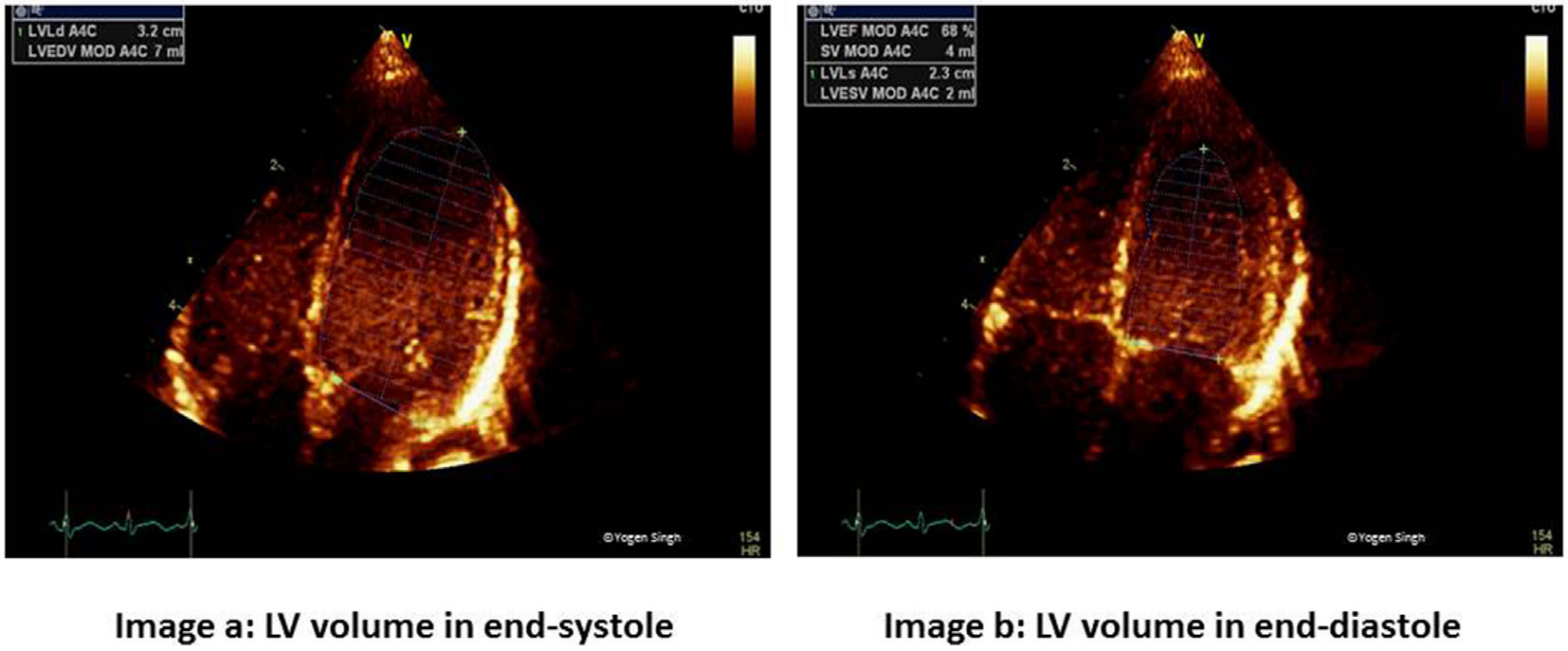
Simpson’s biplane method for measurement of ejection fraction (EF). Image a shows left ventricular end-diastolic volume (LVEDV) and image b shows left ventricular end-systolic volume (LVESV) measurements in apical four-chamber view to calculate EF. Similar measurements are done in apical two-chamber view.
